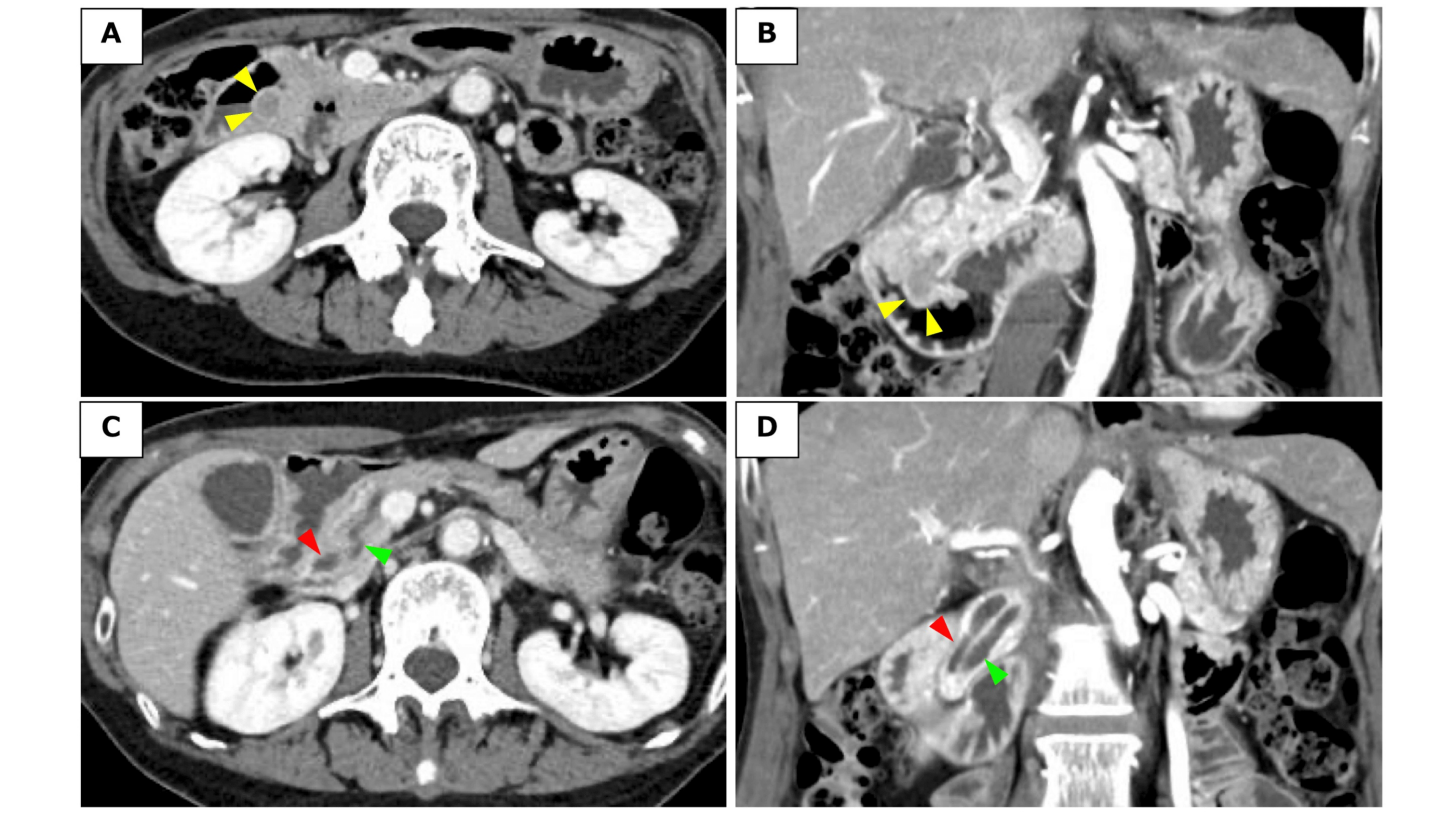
Abdominal computed tomography (CT). (A) Enhanced CT showed a 14mm mass in the ampulla of Vater (arrows) (axial image). (B) Coronal image of the tumor in the ampulla of Vater (yellow arrows). (C) CT scan revealed that the main pancreatic duct (green arrow) and common bile duct (red arrow) were dilated near the ampulla of Vater due to the tumor (axial image). (D) Coronal image of the dilated main pancreatic duct (green arrow) and common bile duct (red arrow).
